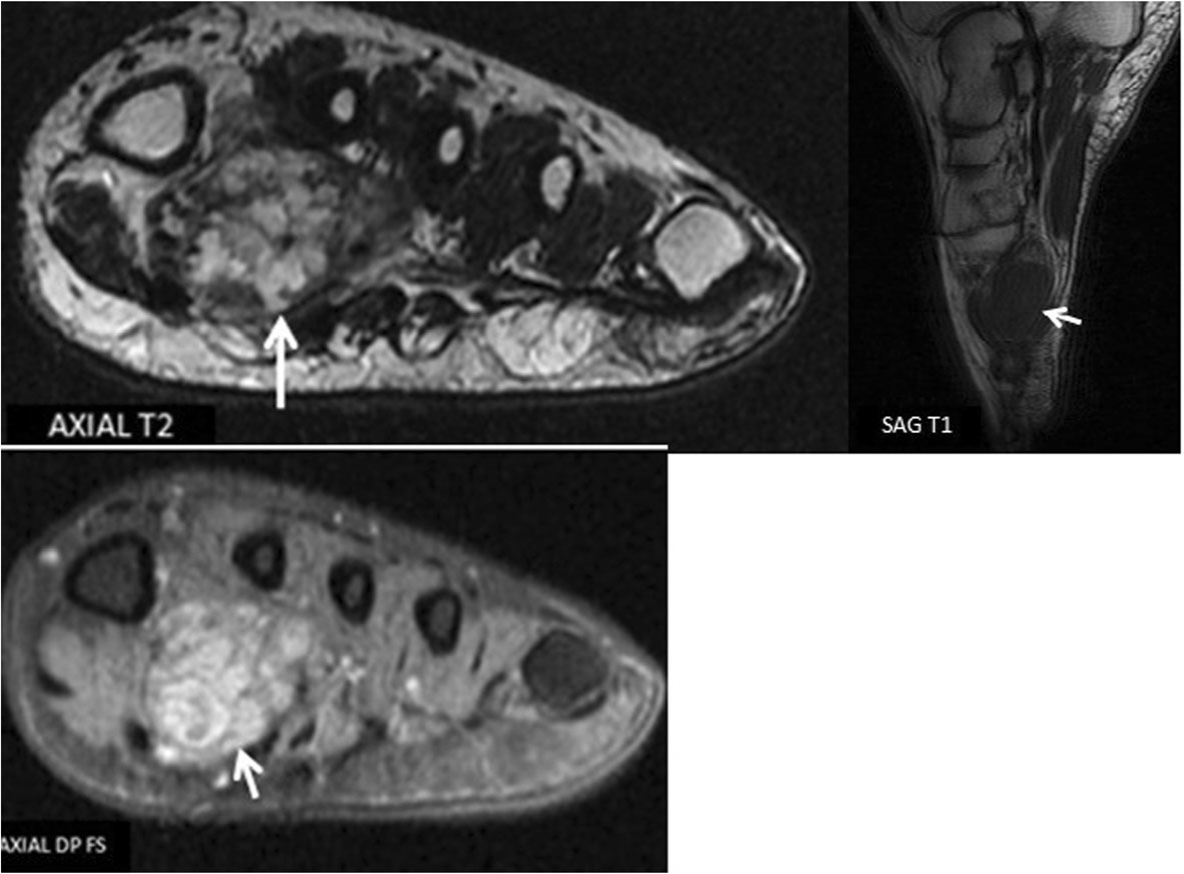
Foot MRI showing a multi-lobulated hemangioma on the plantar side of the foot taking up the first two metatarsals
